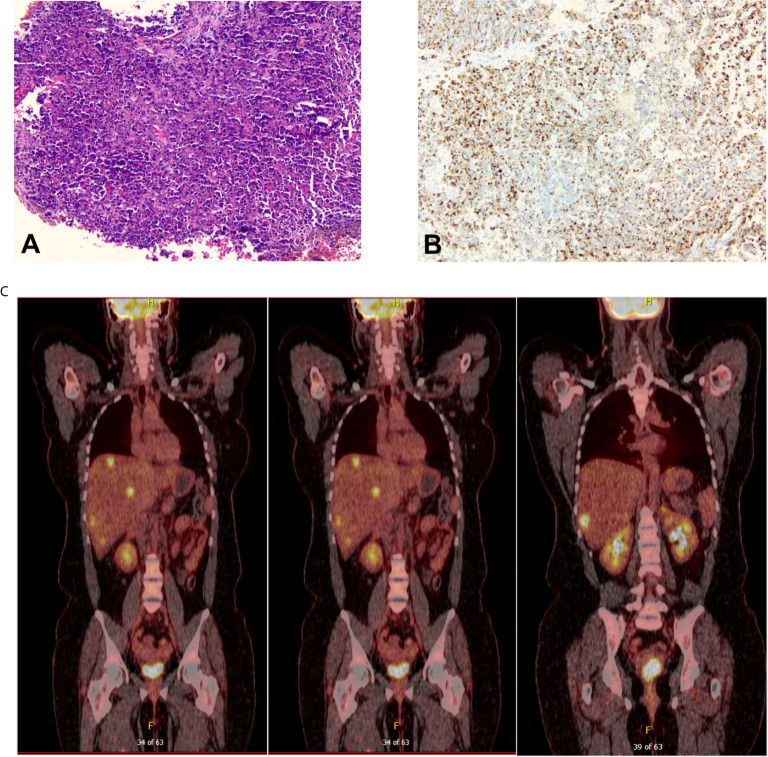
(A) The initial cervical biopsy (100× magnification) demonstrated extensive mitotic activity, high-grade nuclei with hyperchromasia and nuclear molding, scant cytoplasm, and ill-defined cell borders, all characteristic of small-cell carcinoma. (B) The carcinoma was diffusely positive for chromogranin (100× magnification) and synaptophysin (not pictured). (C) Positron Emission Tomography/Computed Tomography (PET/CT) scan at the time of diagnosis that showed evidence of cervical disease, multiple hepatic metastases, and right adrenal gland metastasis.
